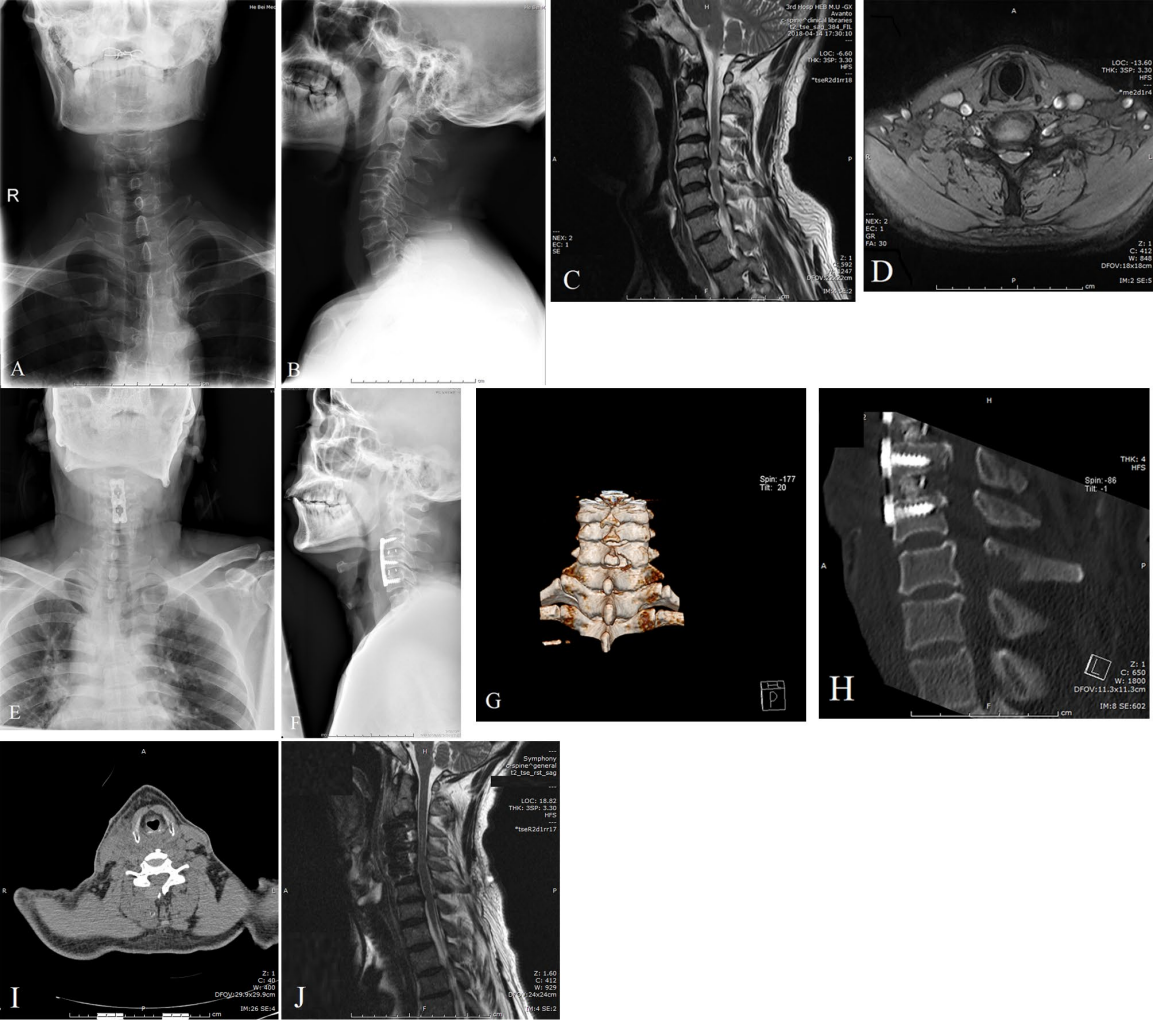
A 52 years old male patient diagnosed with MCSM underwent the hybrid surgery. (A and B) Preoperative anterior and lateral X-ray radiograph of cervical vertebrae. (C) Preoperative MRI indicate the compression from disc herniation (C3-5 and C6-7). (D) Cross-section of MRI at C6-7 level. ((E) and (F)) Anterior and lateral X-ray radiograph of cervical vertebrae after C3-5 ACDF surgery. (G and H) Three-dimensional CT scan of cervical vertebrae after adjunctive PED surgery. (I) Cross-section of CT scan at C6-7 level. (J) Postoperative MRI indicate a sufficient decompression of cervical spinal cord (C3-5 and C6-7)
